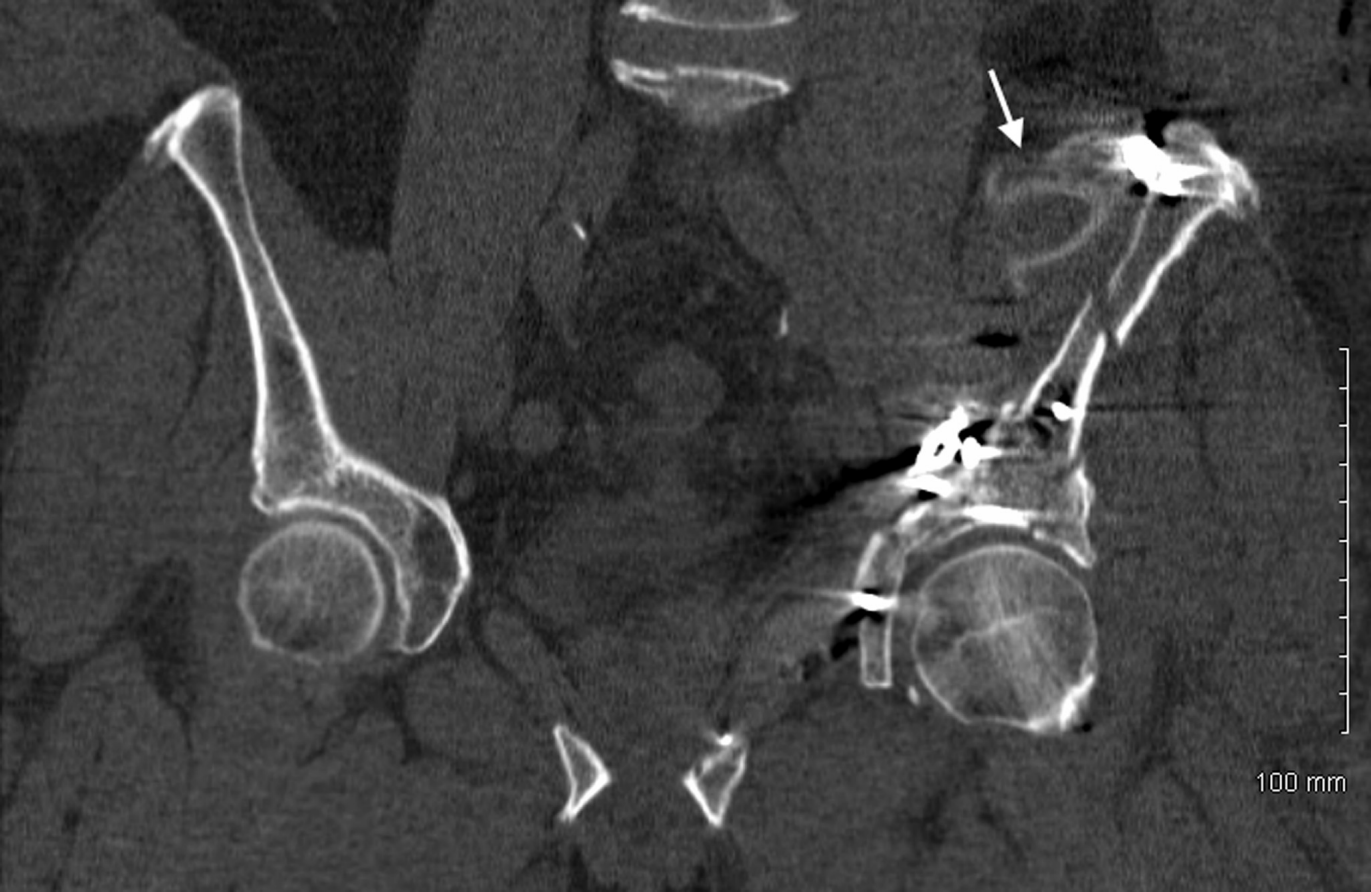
Postoperative axial and coronal pelvic CT scans showing an irregular, hyperdensity (white arrow) in the left iliacus muscle.
